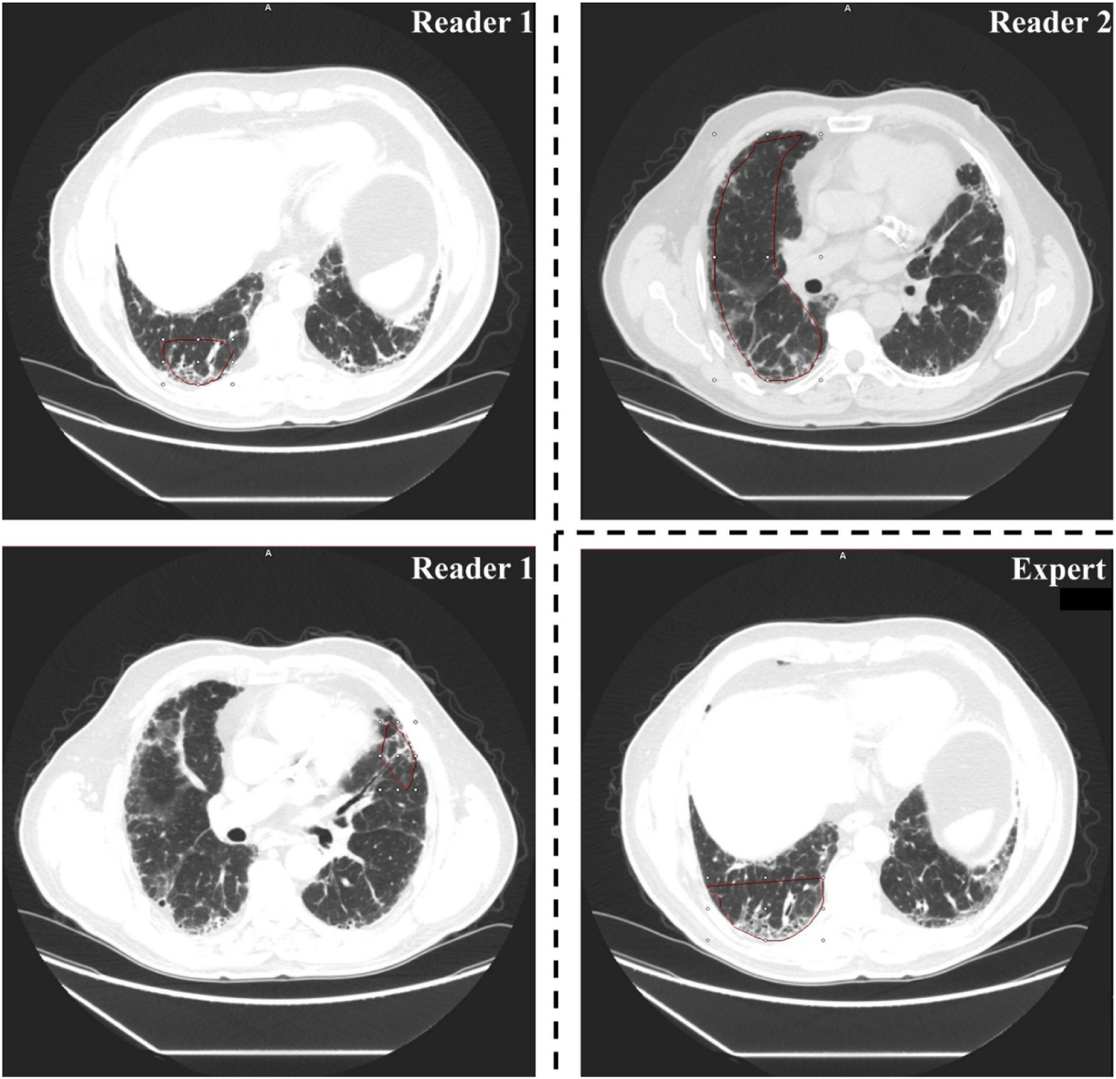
Examples of ROIs used by different users in an identical scan. These images show axial high-resolution computed tomography slices of a patient with an usual interstitial pneumonia pattern. Left: ROIs placed by reader 1. Upper right: ROI placed by reader 2. Bottom right: ROI placed by expert radiologist. ROI, Region-of-interest
